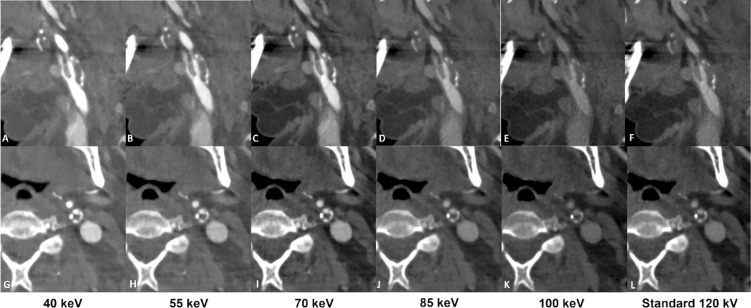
A 76-year-old male suffering from aortic valve stenosis and comorbidities such as diabetes mellitus type 2, and primary arterial hypertension, undergoing PCCT angiography prior to TAVI using 80 ml of 350 mgI/mL Xenetix at an infusion rate of 5.0 ml/second (Xenetix 350 mgI/ml, Guerbet, Villepinte, France). PCCT angiography reconstructions in the parasagittal plane (A-F) and axial plane (G-L) display a subtotal occlusion at the origin of ICA and normal opacification of CCA and ECA. Low-keV VMI + reconstructions ranging from 40 – 70 keV, demonstrate higher iodine attenuation within the perfused lumen and a higher contrast between perfused lumen and soft plaque material compared to the other keV VMI levels and standard CT images. Additionally, beam hardening artifacts are less present in parasagittal reconstructions at low-keV VMI series compared to standard 120 kV images, particularly at 40-55 keV. *CCA* Common Carotid Artery; *ECA* External Carotid Artery; *ICA* Internal Carotid Artery; *PCCT* Photon-counting CT; *TAVI* Transcatheter Aortic Valve Implantation
